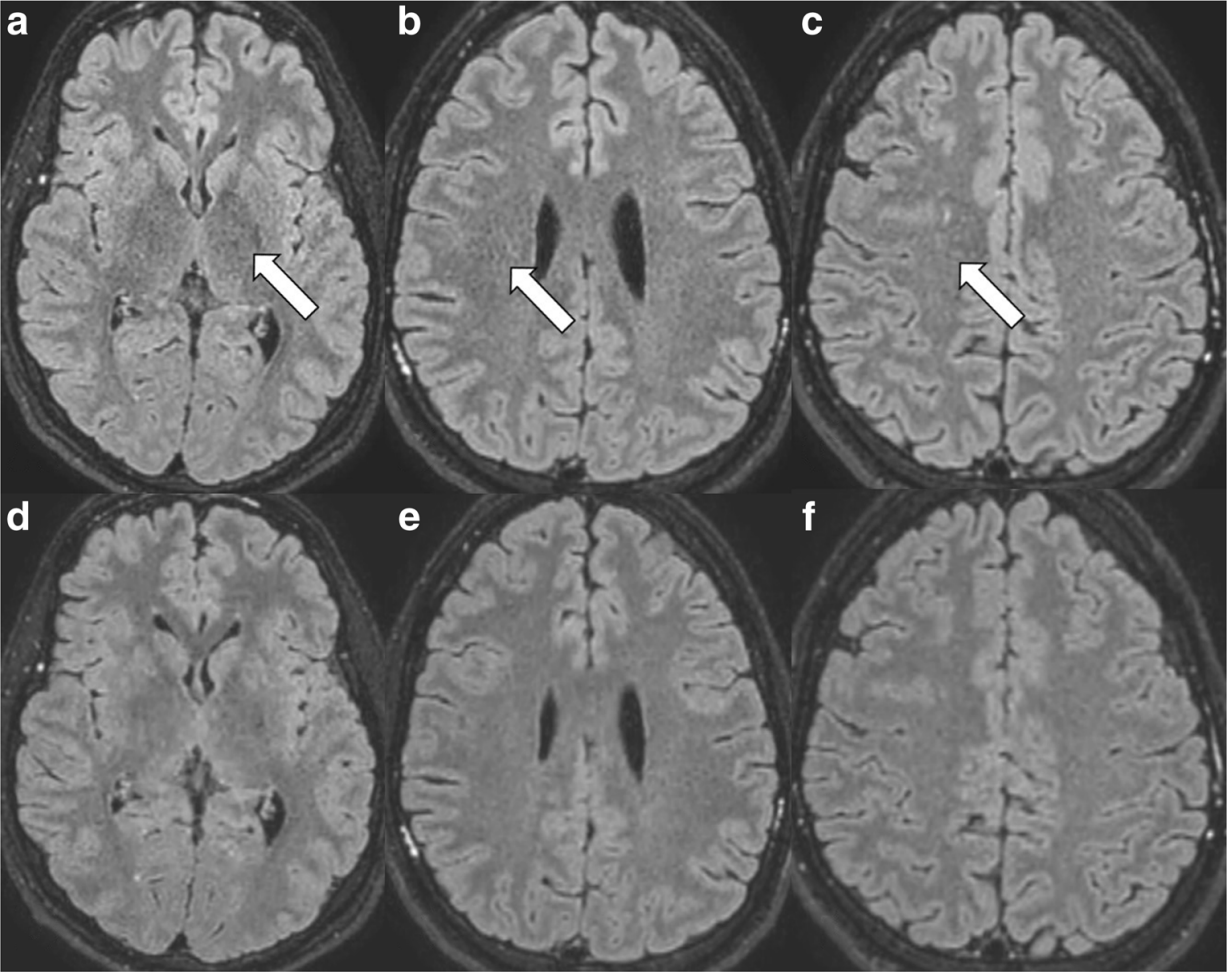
The starry-sky artefact. This artefact was visible as grainy image noise (*white arrows*) on the transverse reconstructions of the 3D FLAIR sequence with Compressed SENSE (a-c) if compared to corresponding transverse reconstructed images of a 3D FLAIR with Compressed SENSE but without this artefact in the same volunteer (d-f) after sequence repetition. The artefact was also visible on coronal reconstructions and on sagittal source images (not depicted) of the 3D FLAIR sequence
